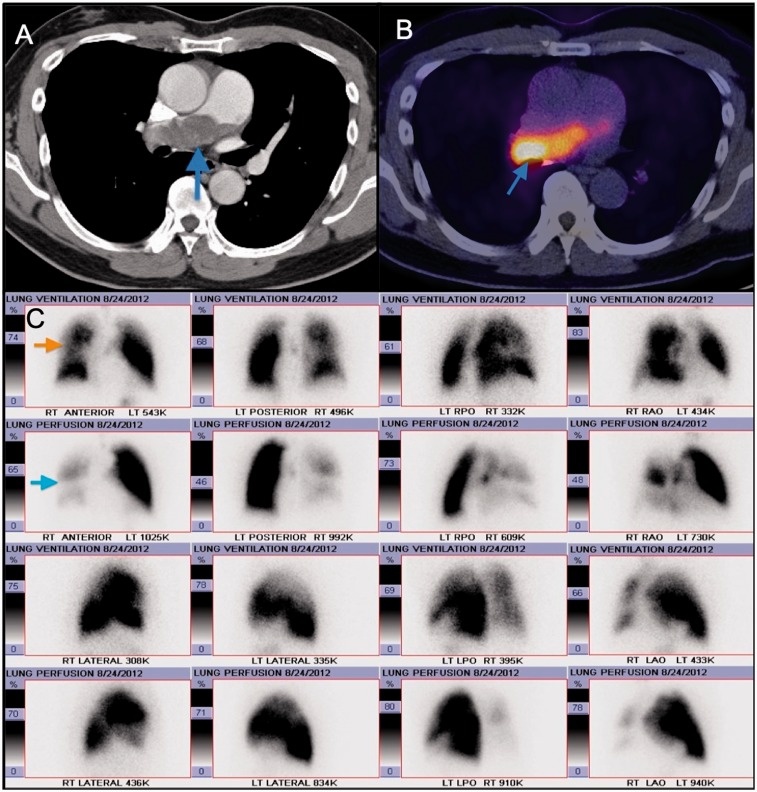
Panel A and B: Axial contrast enhanced CT mage showing a large
